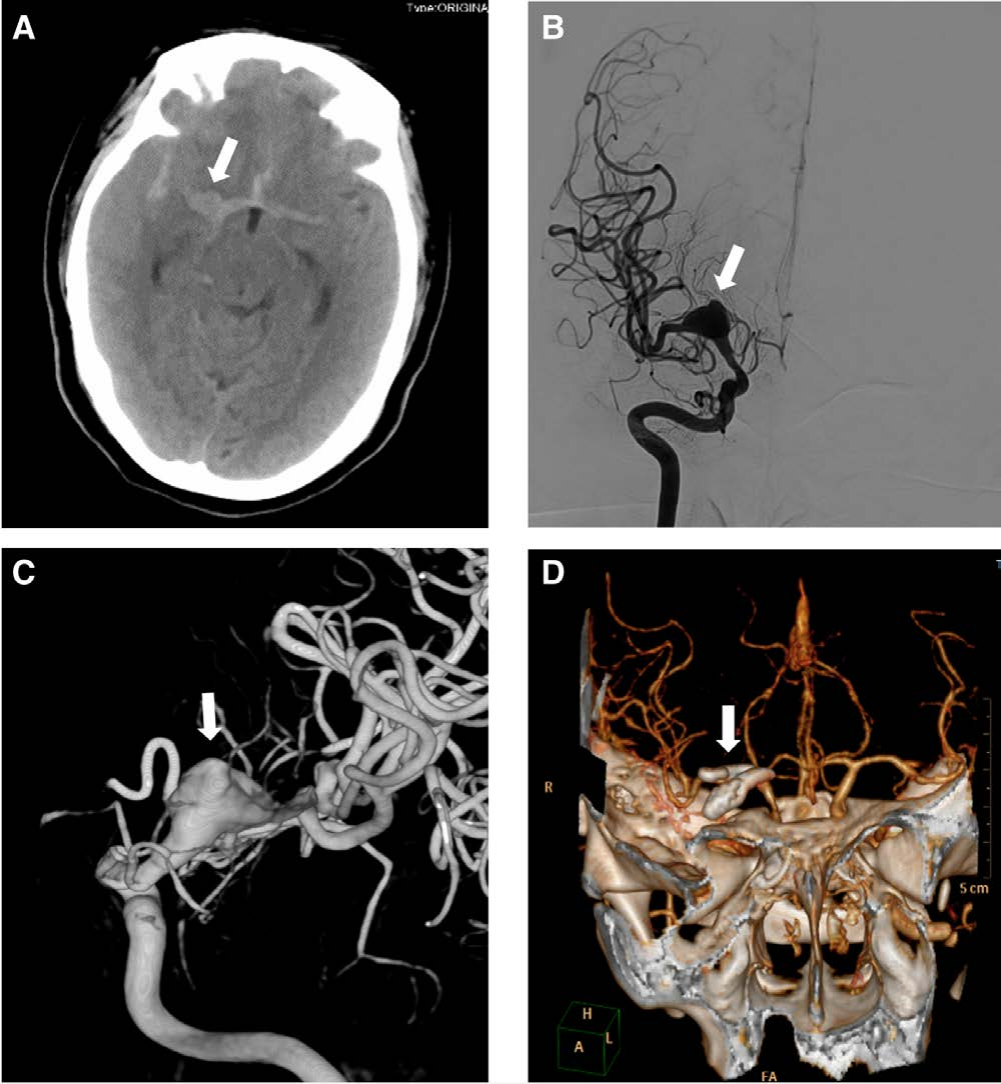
Neurosurgical clipping for the treatment of a ruptured aneurysm in the right middle cerebral artery (R-MCA) (A) Non-contrast CT scan showing subarachnoid hemorrhage and suspected R-MCA aneurysm. (B–C) Digital subtraction angiography images confirming recurrent of large spindle-shaped aneurysm in R-MCA. (D) Postoperative CTA examination showing complete clipping of R-MCA aneurysm. CT = computed tomography.
